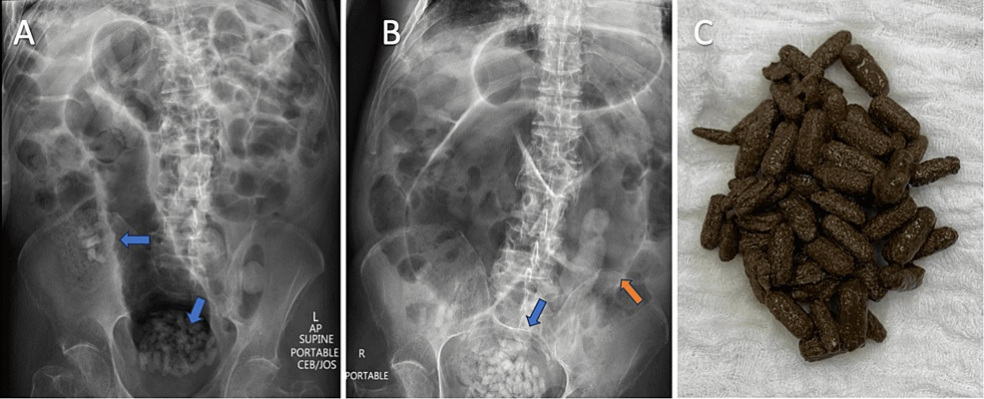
Radio-opaque densities identified on plain radiograph and associated large bowel distention. A. Nonobstructive bowel gas pattern with air distention of the sigmoid colon. Retained radiopaque bodies in the rectal ampulla and right upper quadrant (blue arrows). B. Progression of air distention of the sigmoid colon (orange arrow), fecal impaction, and redemonstration of radio-opaque densities within the rectum (blue arrow). C. Removed pharmacobezoar through colonoscopy.
